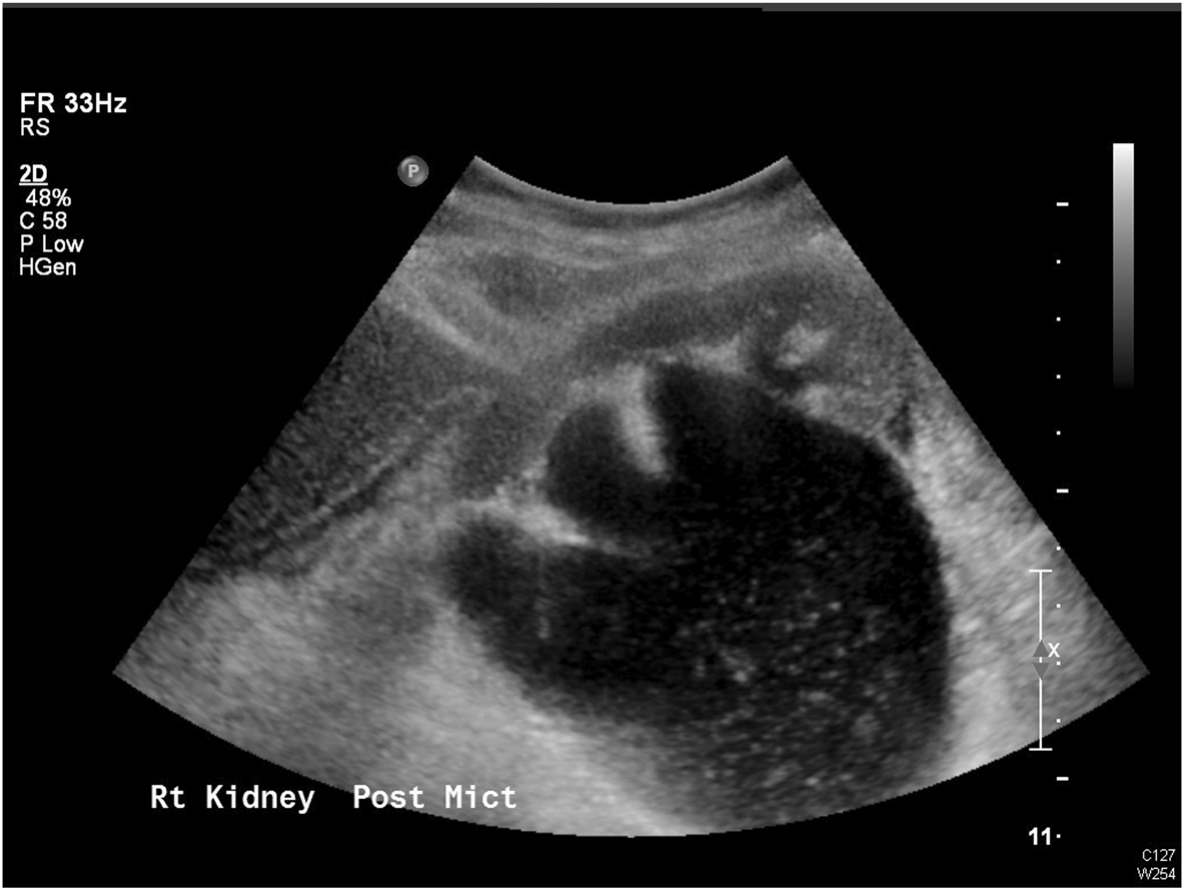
Persisting hydronephrosis on ultrasound with debris.
